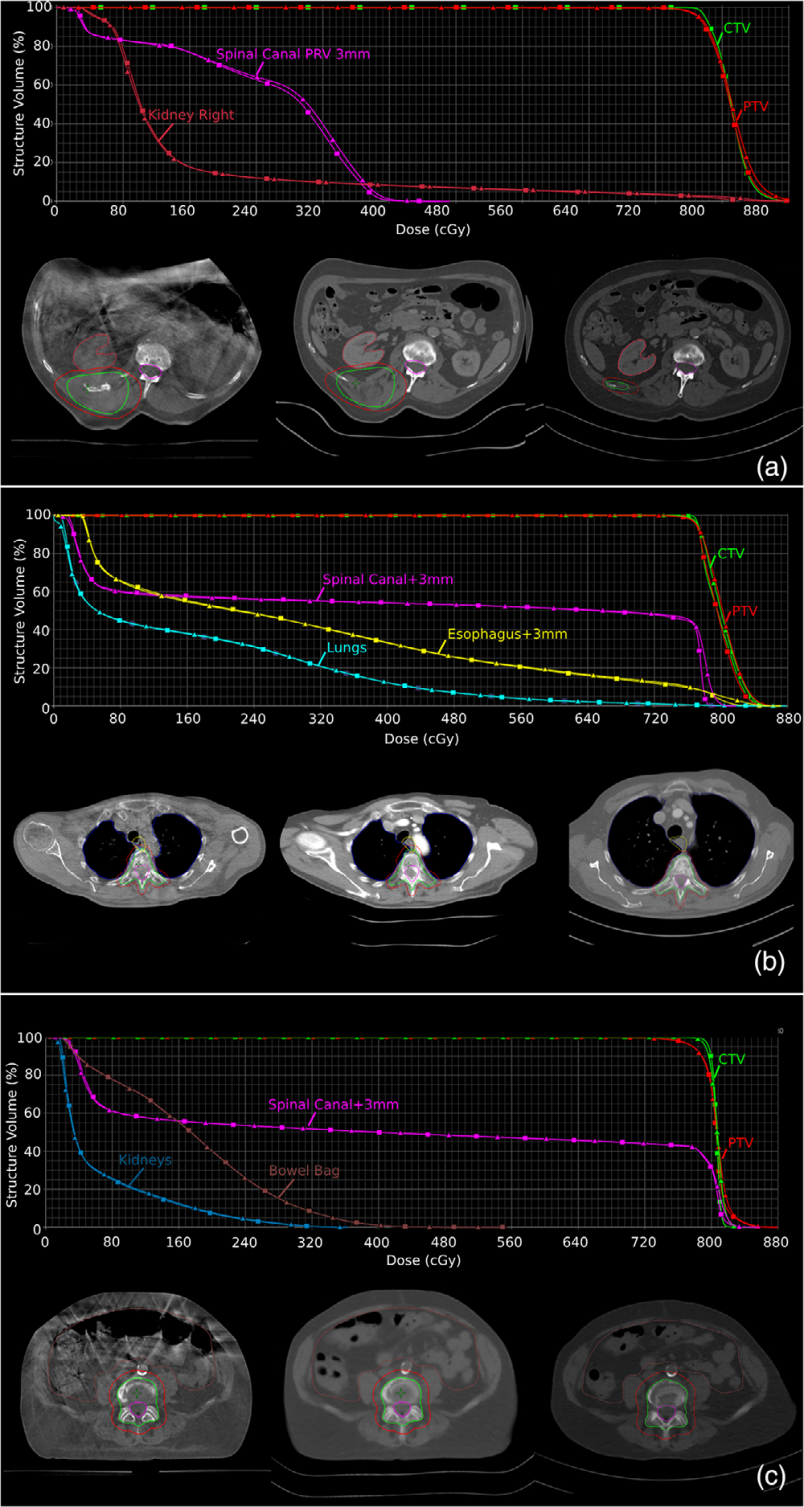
Examples of anatomical differences among the cone‐beam CT, synthetic CT, and diagnostic CT (from left to right, respectively). In addition, for each patient a comparison of the dose–volume histogram between the synthetic CT (square) and the cone‐beam CT (triangle) is shown. Part (a) shows patient 12, (b) patient 4, and (c) patient 9.
